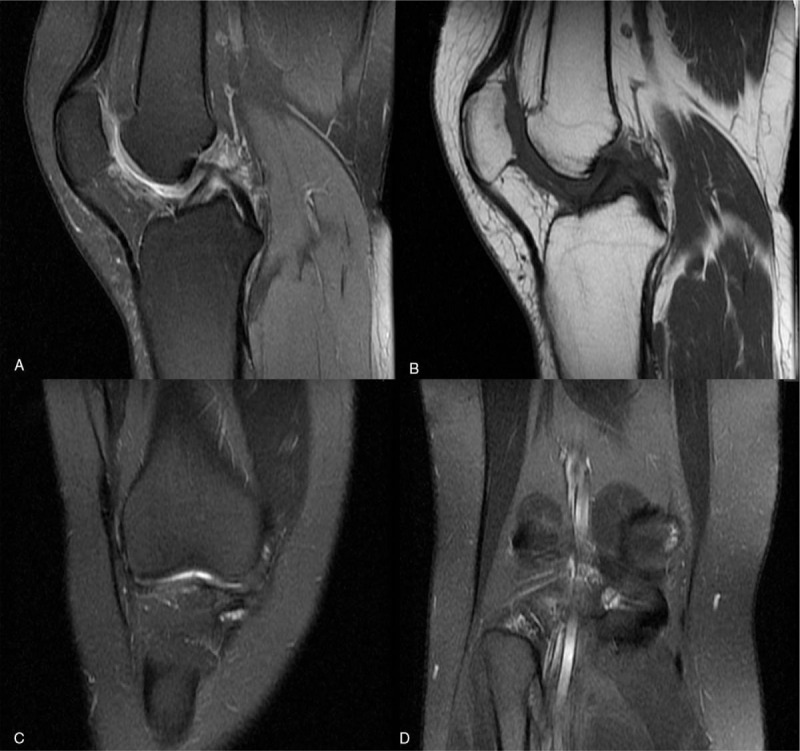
Follow-up MRI performed 13 mo after the second surgery. (A) Sagittal MRI T2WI and (B) Sagittal MRI T1WI sequences did not show recurrence. (C, D) Coronal MRI T2WI sequence did not indicate recurrence. MRI = magnetic resonance imaging.
